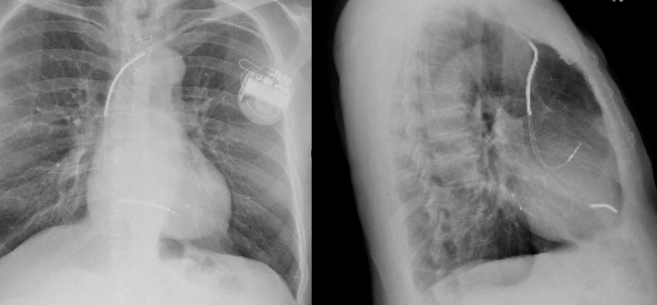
2-View Chest X-Ray Reveals all 3 Leads in Place Including the Bipolar Left Ventricular Lead, Which Is the Posterolateral Branch
